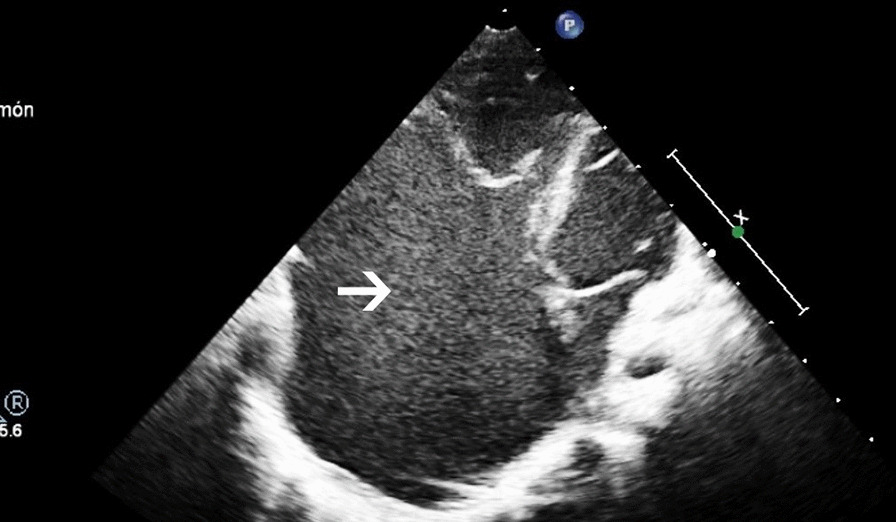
Neonatal echocardiography, 4-chamber plane. The arrow points the right atrial dilatation
